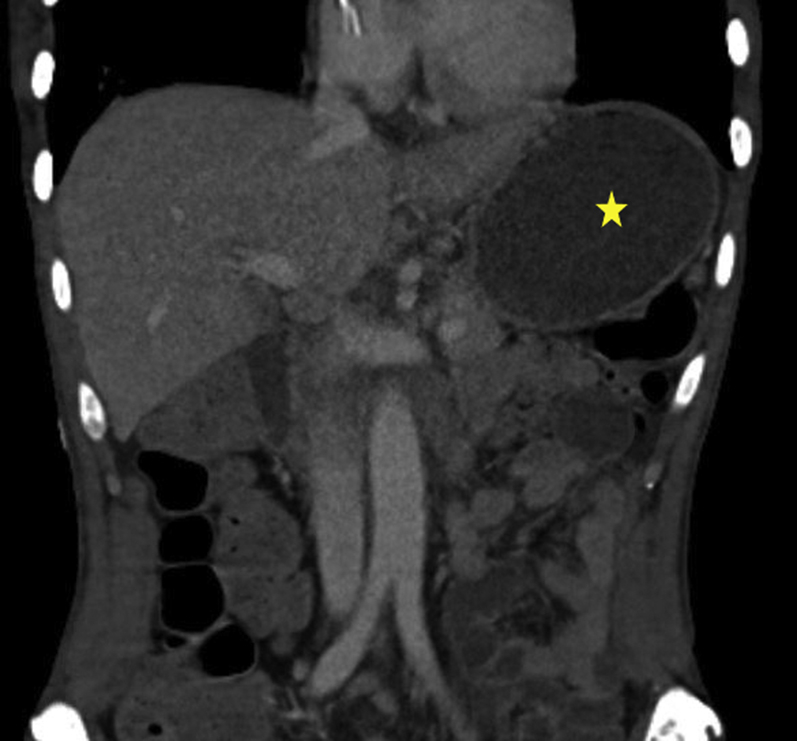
CT scan of abdomen showing a distended gastric pouch (*yellow asterisk*) secondary to gastric outlet obstruction.
